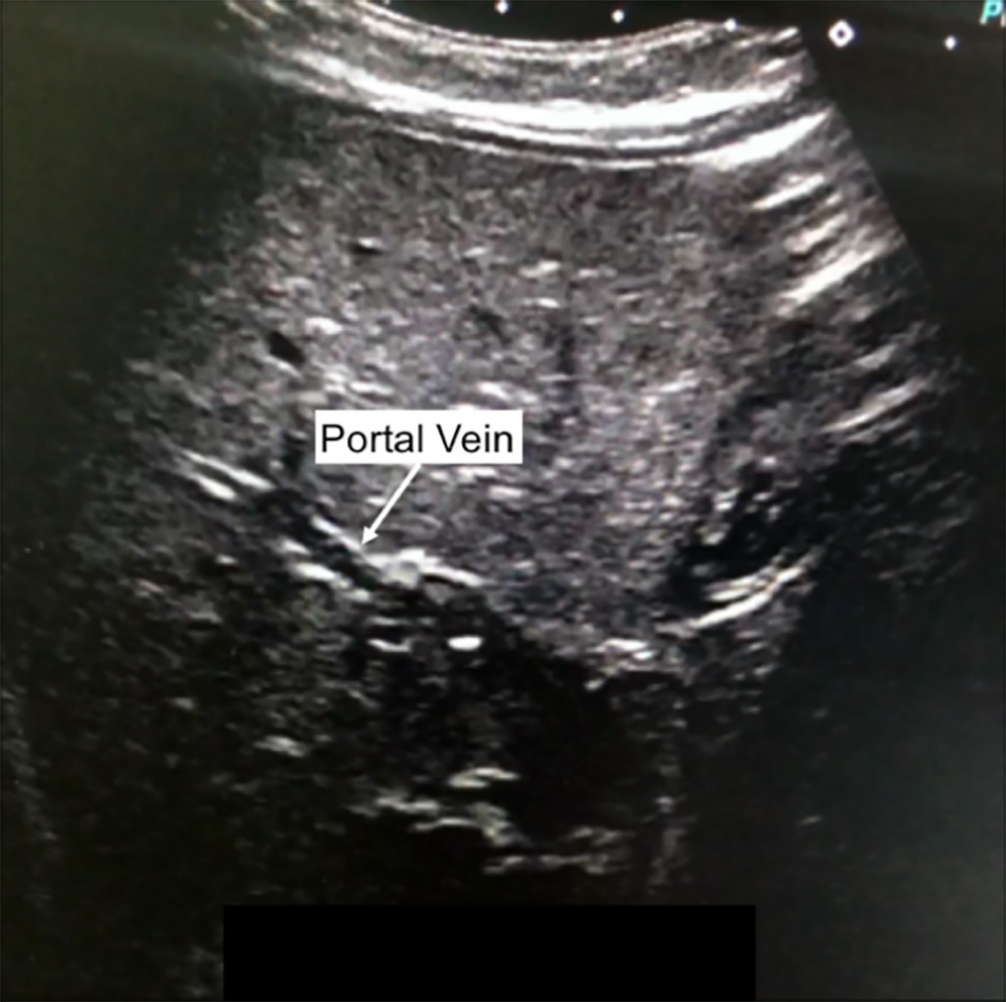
VIDEO 1. A Video clip of liver ultrasound images. Transverse imaging of the liver captured air bubbles in the portal vein.
